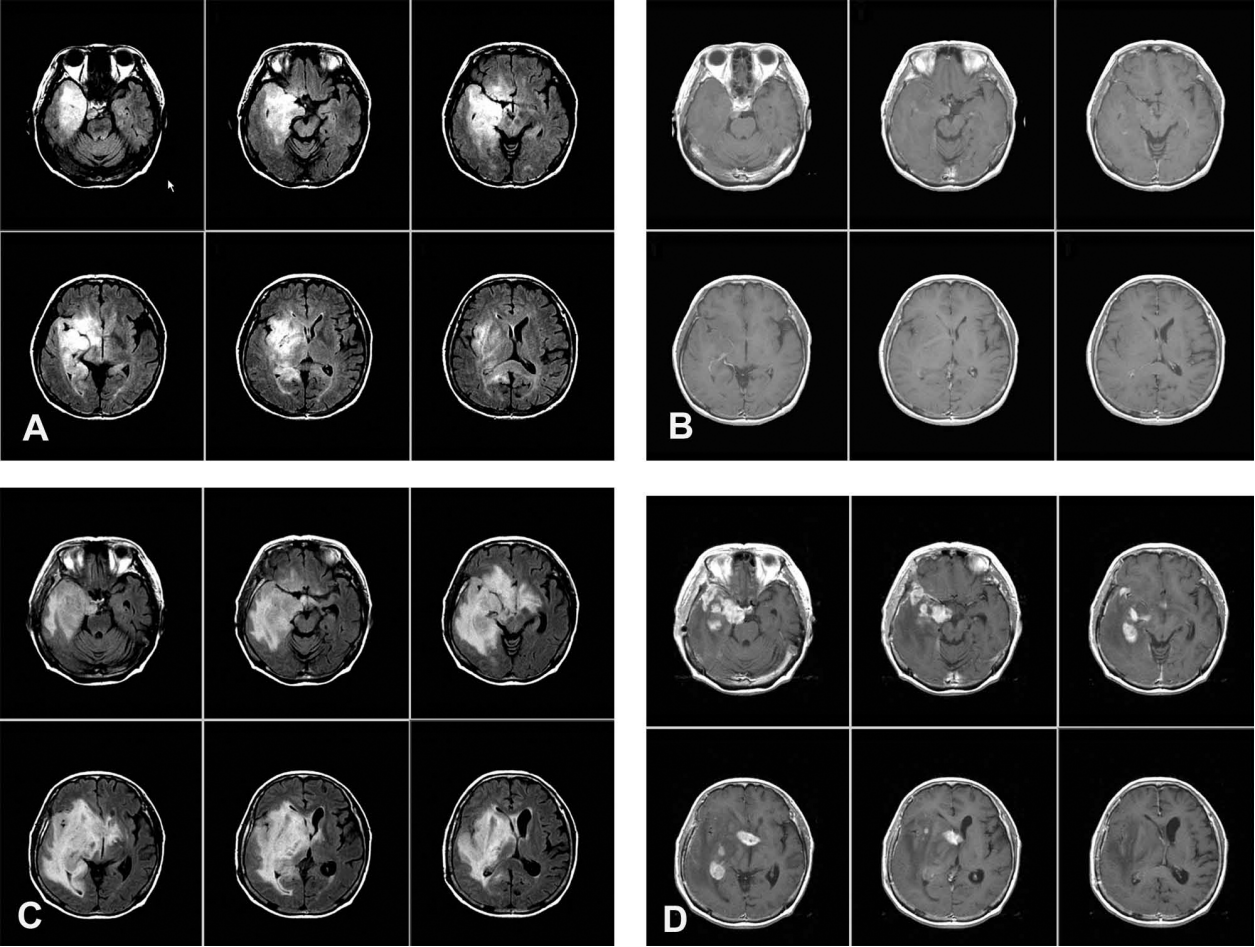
Serial MR images of gliomatosis cerebri. Case 2: (A & B) at the initial presentation, highly infiltrated lesion is found on the FLAIR image, but there is no enhancing lesion on the T1 weighted enhanced image (T1WI), (C & D) After 3 months, the tumor is widely spread on the FLAIR images and new contrast-enhancing lesions diffusely appears on the T1WI.
